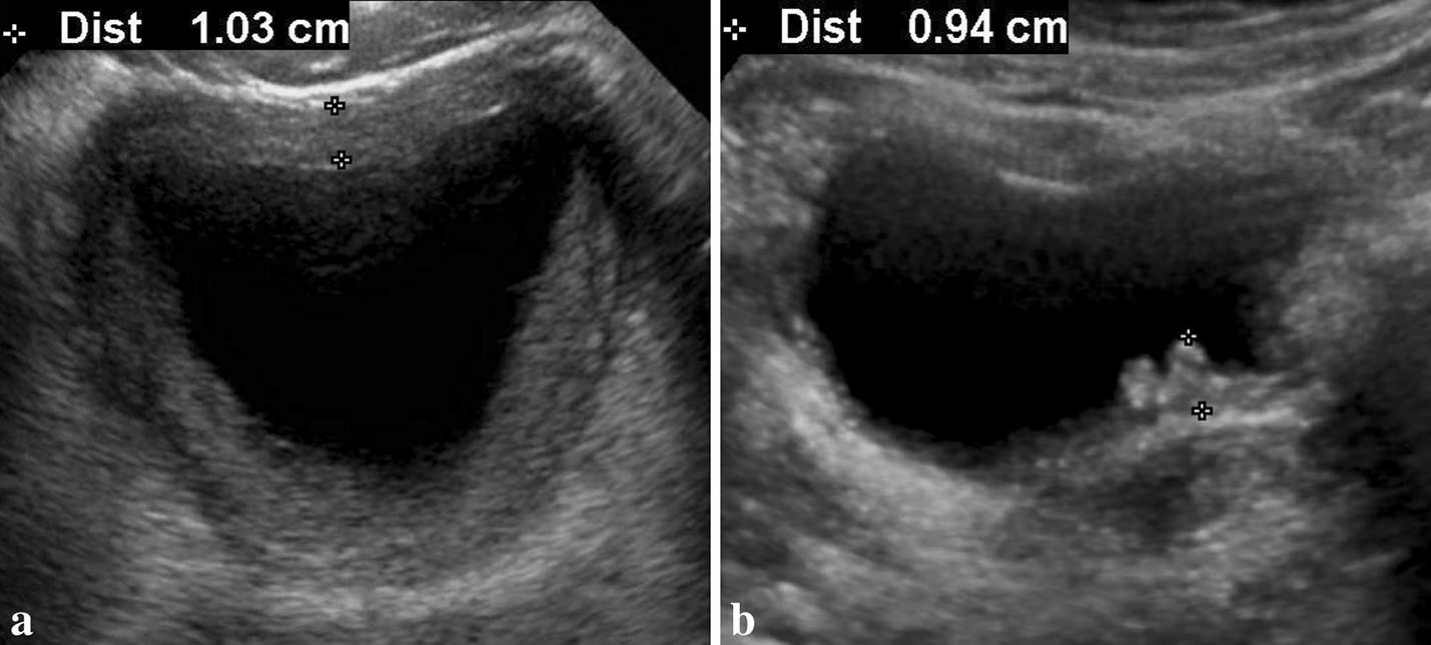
Two types of bladder wall thickening on bladder sonography of patients with hemorrhagic cystitis. a Diffuse type wall thickening; continuous, circumferential and even wall thickening of bladder. b Nodular type wall thickening; more than 3 mm-height of nodular protruding focus in a background of either continuous or discontinuous wall thickening of bladder
